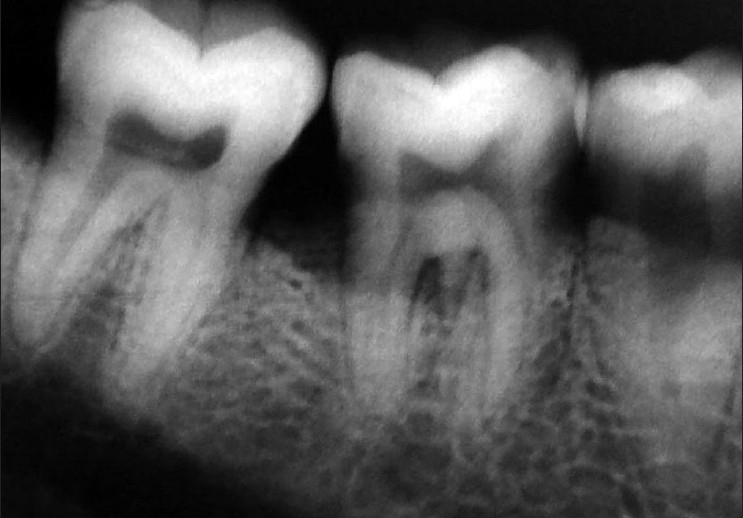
Preop radiograph (right side)
